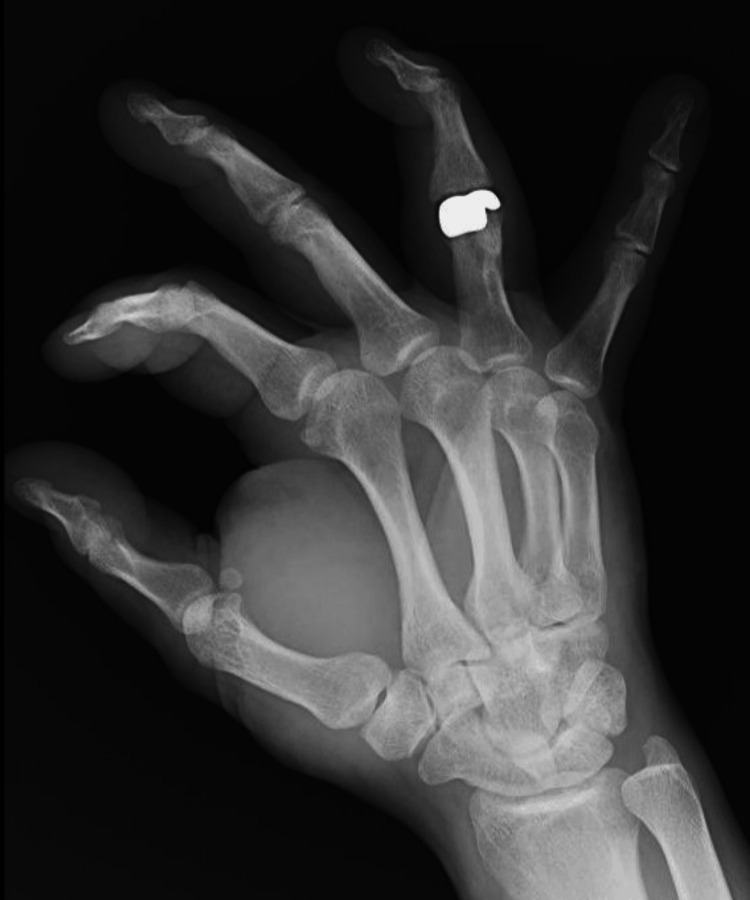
Lateral X-ray obtained eight months after surgery
